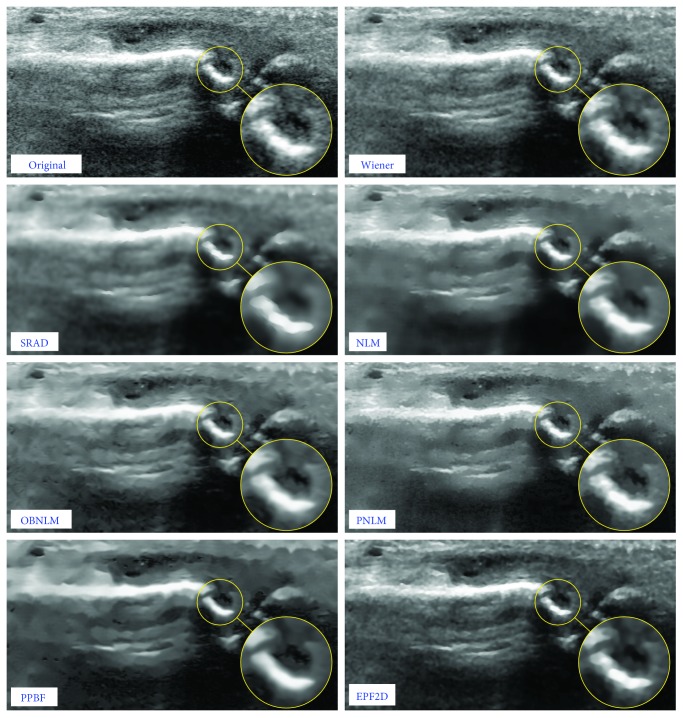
Visual comparison of the filtering efficiency evaluated on a real ultrasound image contaminated by multiplicative noise.
